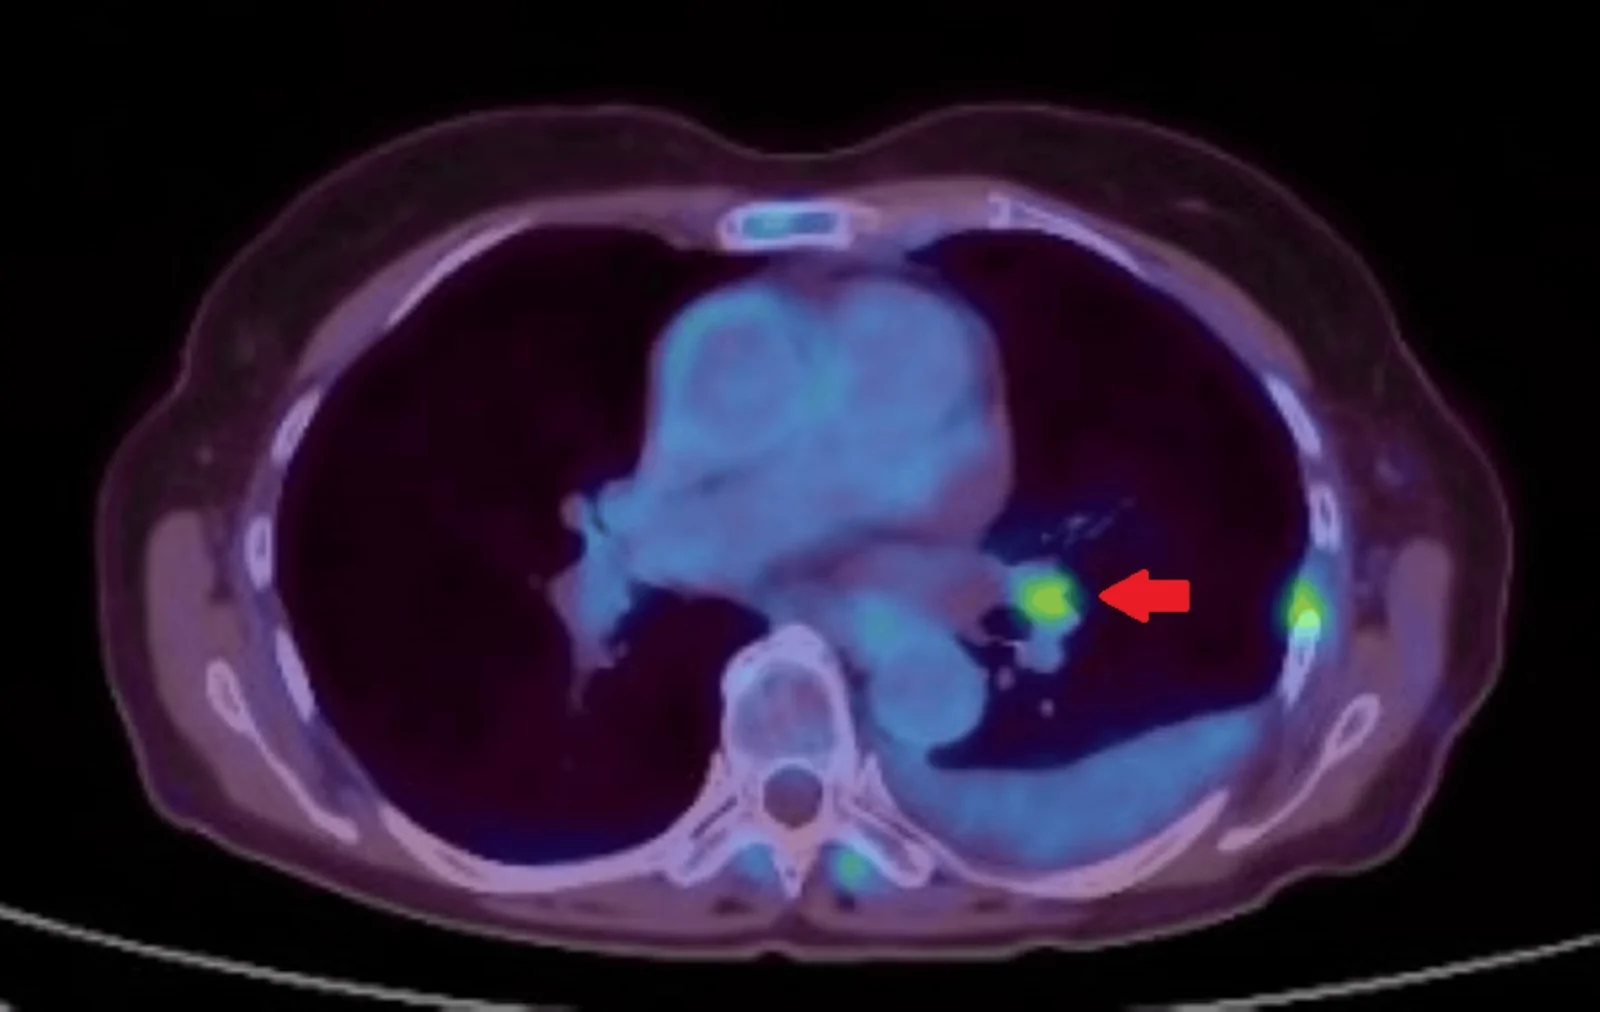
FDG chest PET-CT findings FDG PET-CT showing increased FDG uptake in the left mediastinal lymph node. The findings were suggestive of lymphomatous lymph node involvement. FDG: fluorodeoxyglucose; PET-CT: positron emission tomography-computed tomography
